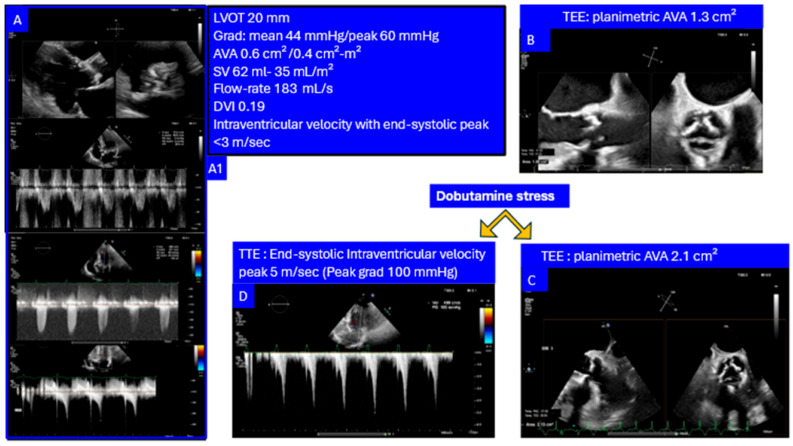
Echocardiographic evaluation of high TVG suggesting severe aortic stenosis with concomitant inducible dynamic intraventricular obstruction Dobutamine stress induced a marked rise in end-systolic intraventricular velocity (5 m/s, peak gradient 100 mmHg), with TEE planimetry confirming functional valve area reserve. (A): baseline TT examination (see (A1) box). (B): Baseline TEE. Dobutamine stress test (C): TEE showing increase in AVA; (D): Doppler TT showing an impressive intraventricular gradient).
